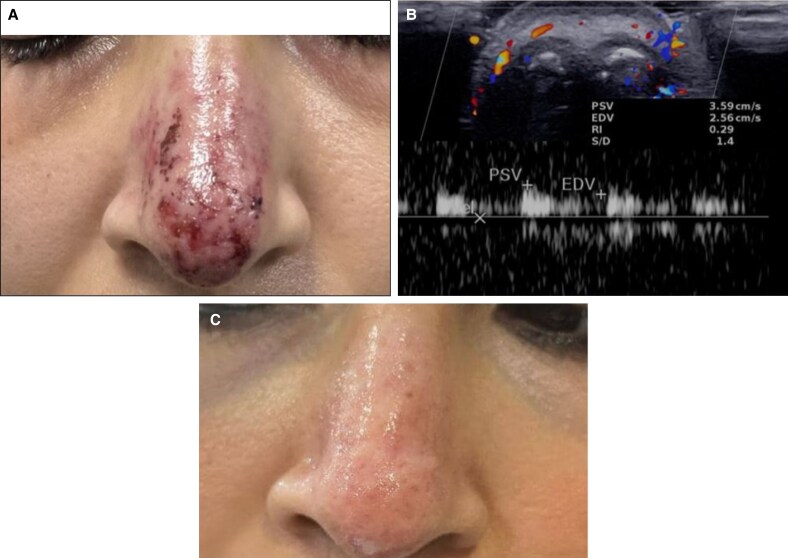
Clinical and ultrasound follow-up 2 days and 1 week after hyaluronidase treatment (Case 2, a 32-year-old woman). (A) Resolution of clinical symptoms 2 days posttreatment. (B) Transverse color Doppler ultrasound showing restored flow. (C) Clinical image at 1-week follow-up.
